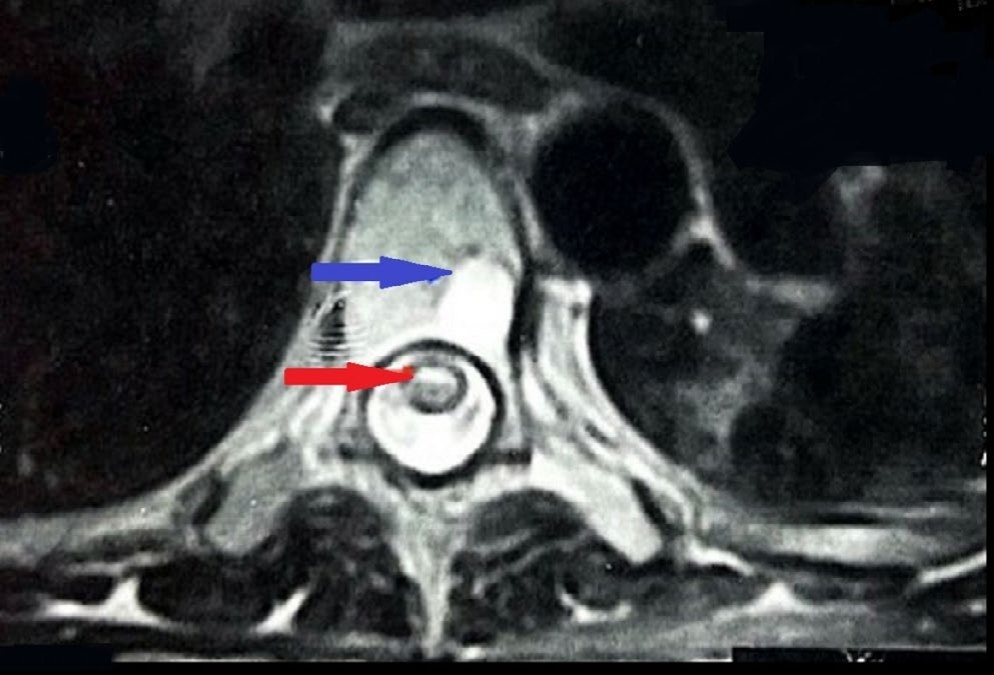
The patient's magnetic resonance imaging, transverse view of the sixth thoracic vertebra, showing both syringomyelia (red arrow) and the body lytic lesion (blue arrow).
